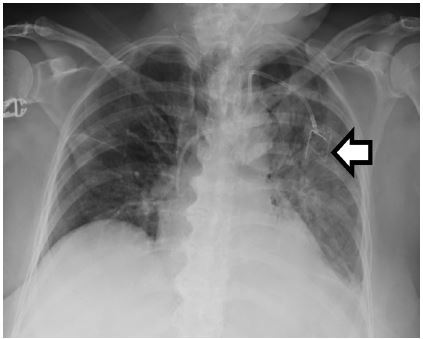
Chest radiograph of febrile cancer patient with left-sided chemotherapy port in place.
